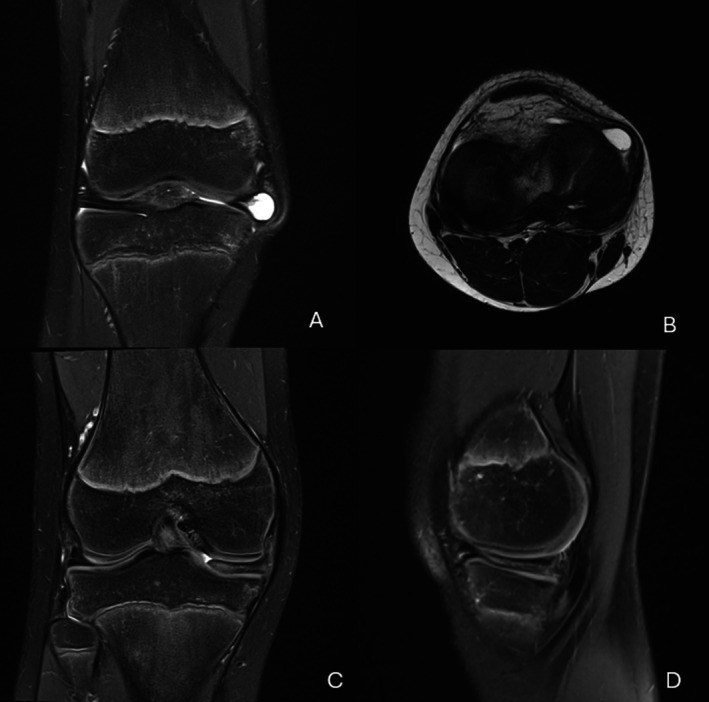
MRI of the right knee. The coronal T2‐weighted scan shows a parameniscal cystic mass from the medial meniscus (A) The axial T2 shows the parameniscal cystic lesion protruding from the junction of the pars intermedia and the anterior horn, extending through the medial retinaculum at the junction of the medial collateral ligament (B) Both the coronal and sagittal scans show the medial discoid meniscus (C, D).
